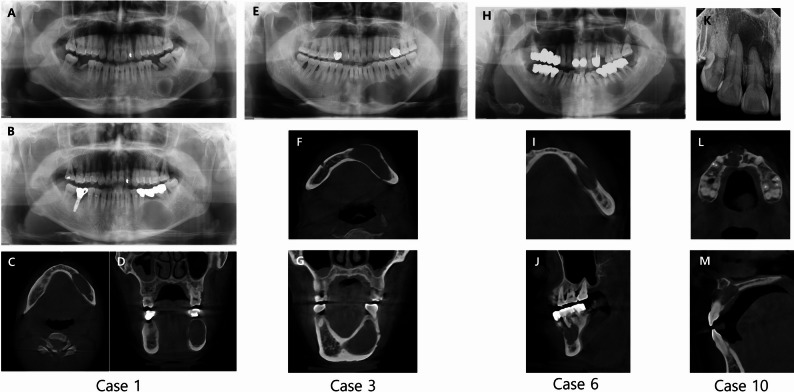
Case 1(A ~ D): A A Panoramic radiograph showed a well-defined, round-shaped unilocular lesion was observed in the left posterior mandible. B A follow-up panoramic image taken 12 years later showed that the lesion had more than doubled in size. C, D CBCT revealed buccolingual cortical thinning and expansion. Case 3(E ~ G): E A Panoramic image revealed well-defined radiolucent lesion with a scalloped margin was observed in the left mandibular anterior to posterior region. F, G CBCT revealed buccolingual cortical thinning and expansion. Case 6(H ~ J): A moderately defined radiolucent lesion was observed in the apical area of teeth #35 and #36, which had previously undergone endodontic treatment. Case 10(K ~ M): A moderately defined scalloped radiolucent lesion with slight labiopalatal cortical thinning was observed in the maxillary anterior region
